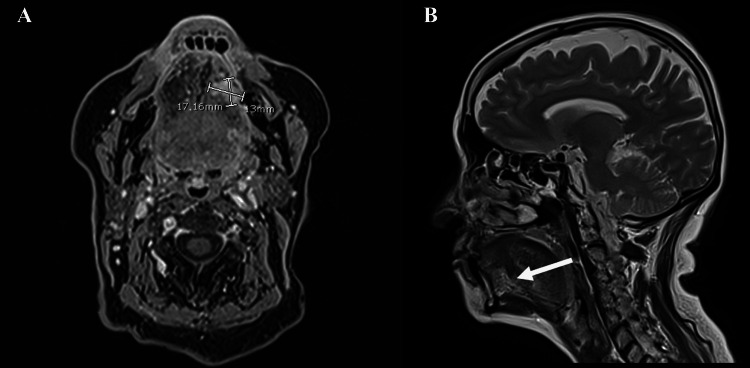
MRI of head and neck: (A) T1 sequence contrasted in the axial plane and (B) T2 sequence in the sagittal plane Image (A) shows an axial MRI scan revealing a poorly defined lesion on the left lateral border of the tongue, with intense post-contrast enhancement. The lesion measures 11 × 13 × 17 mm in the longitudinal, anteroposterior, and transverse axes, respectively. Image (B) shows the same lesion in the sagittal plane (indicated by the white arrow). MRI: Magnetic resonance imaging
